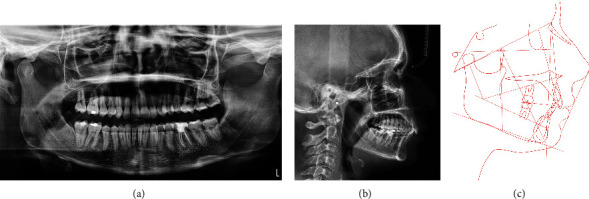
(a) Final panoramic X-ray, (b) teleradiograph, and (c) cephalometry.
